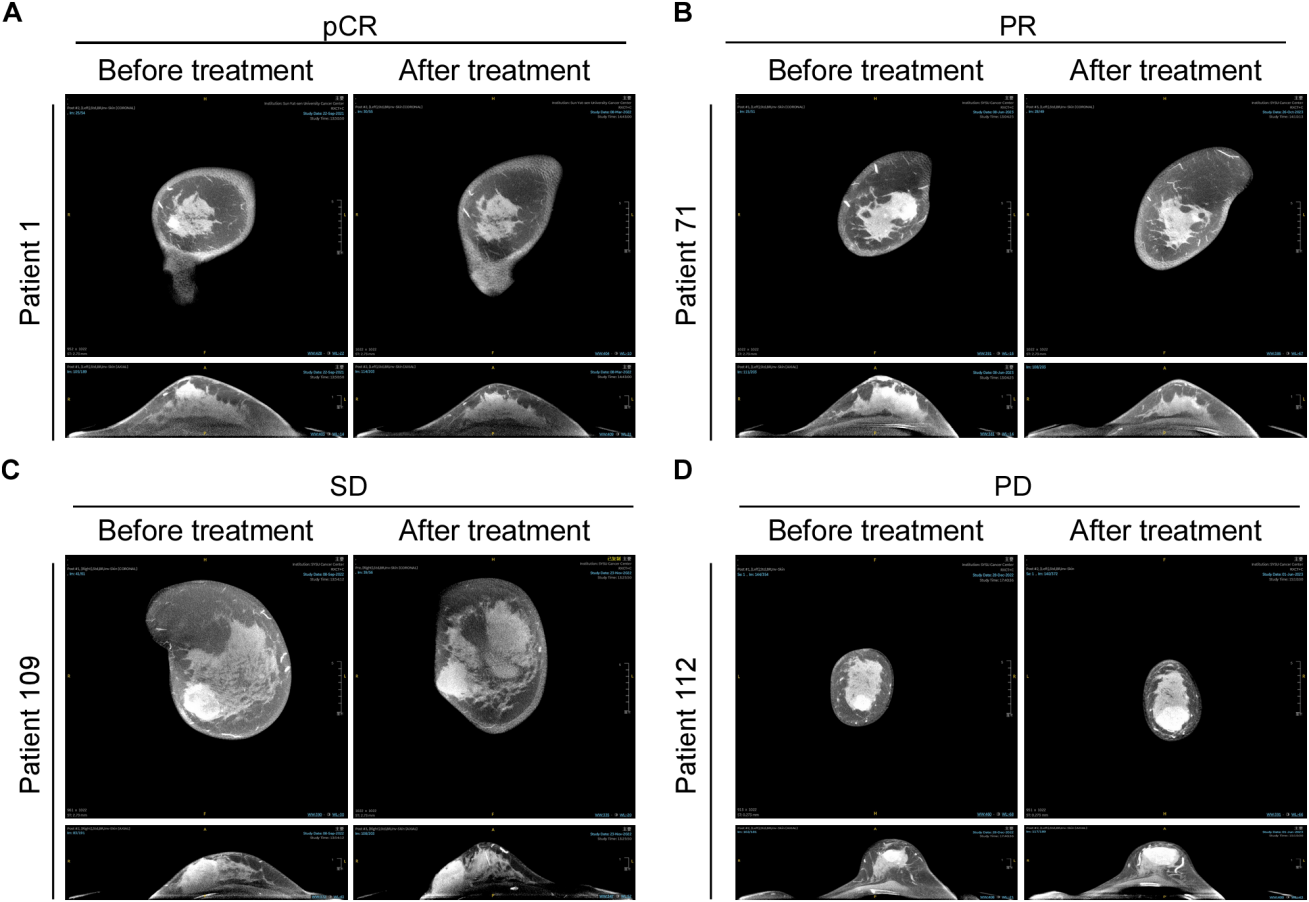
CT Images of representative patients before and after neoadjuvant chemoimmunotherapy (nCIT), demonstrating radiological responses according to RECIST 1.1 Criteria (A–D) Representative CT images showing pathological complete response (pCR), partial response (PR), stable disease (SD), and progressive disease (PD) in early-stage triple-negative breast cancer patients before and after treatment.
